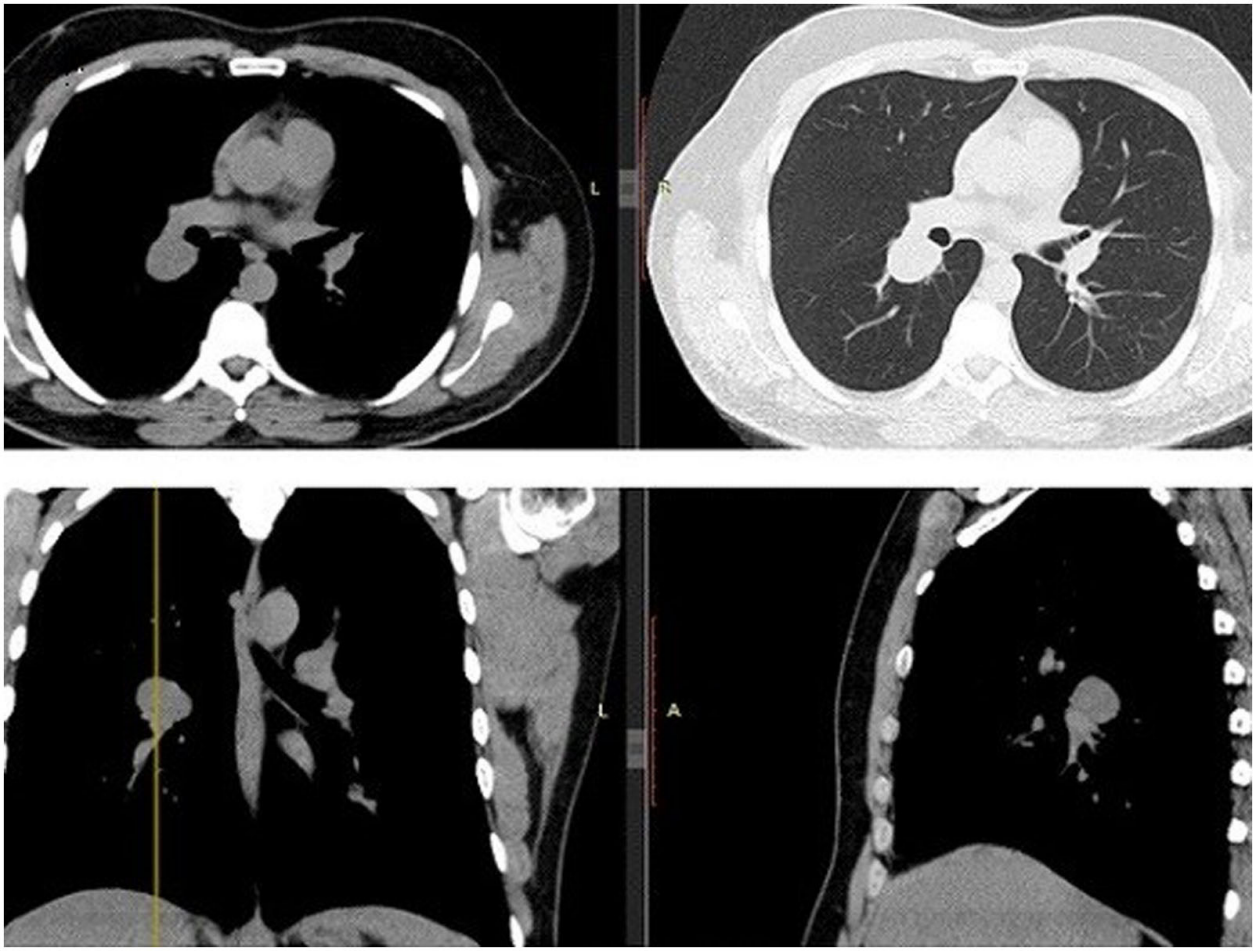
Chest CT. Transverse/coronal/sagittal planes demonstrate a round homogenous mass 32 mm × 27 mm in size in the superior segment of right lower lobe.
